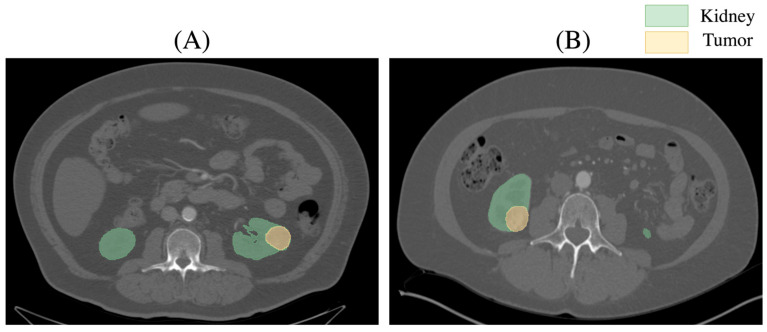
Representative axial CT slice from the KiTS dataset depicting a renal tumor and surrounding kidney anatomy. The tumor is located at the lower pole of the left kidney in (A) and at the lower pole of the right kidney in (B).
